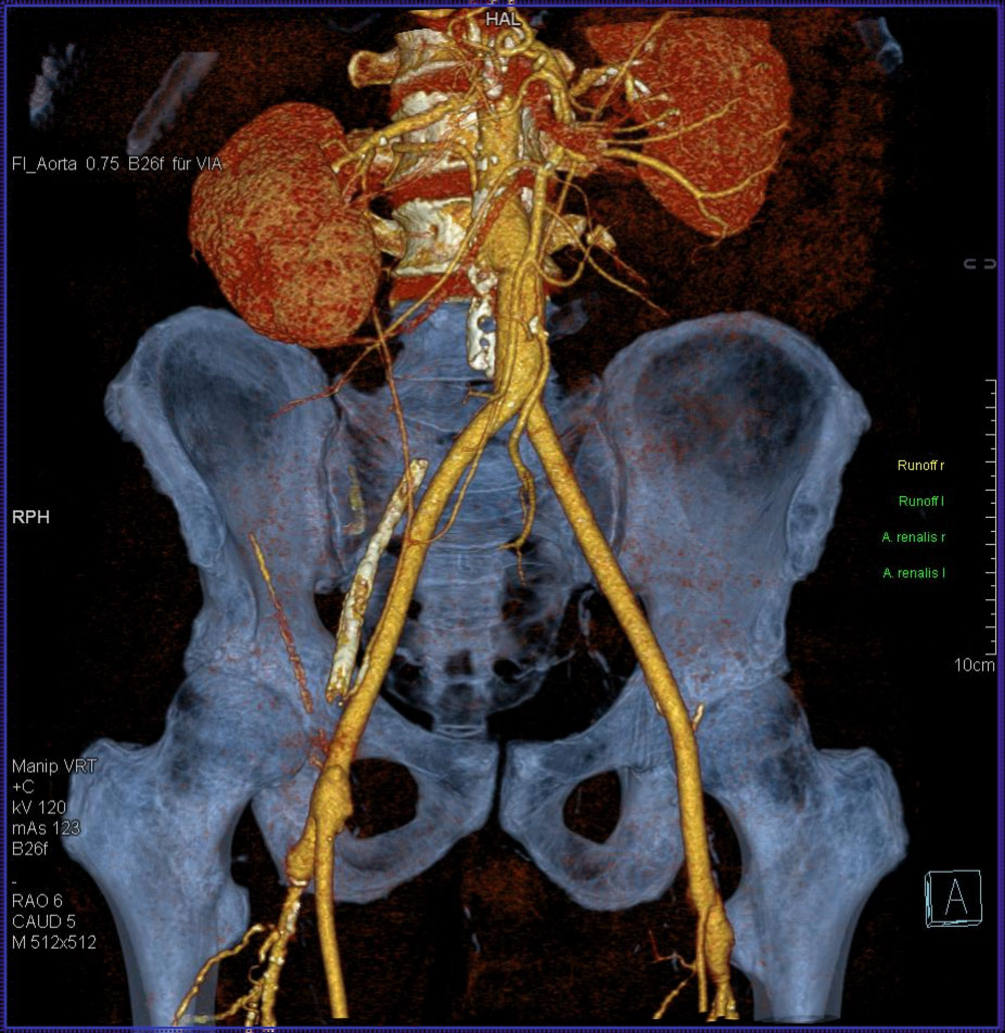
The graft appears normal on postoperative follow-up computed tomography
